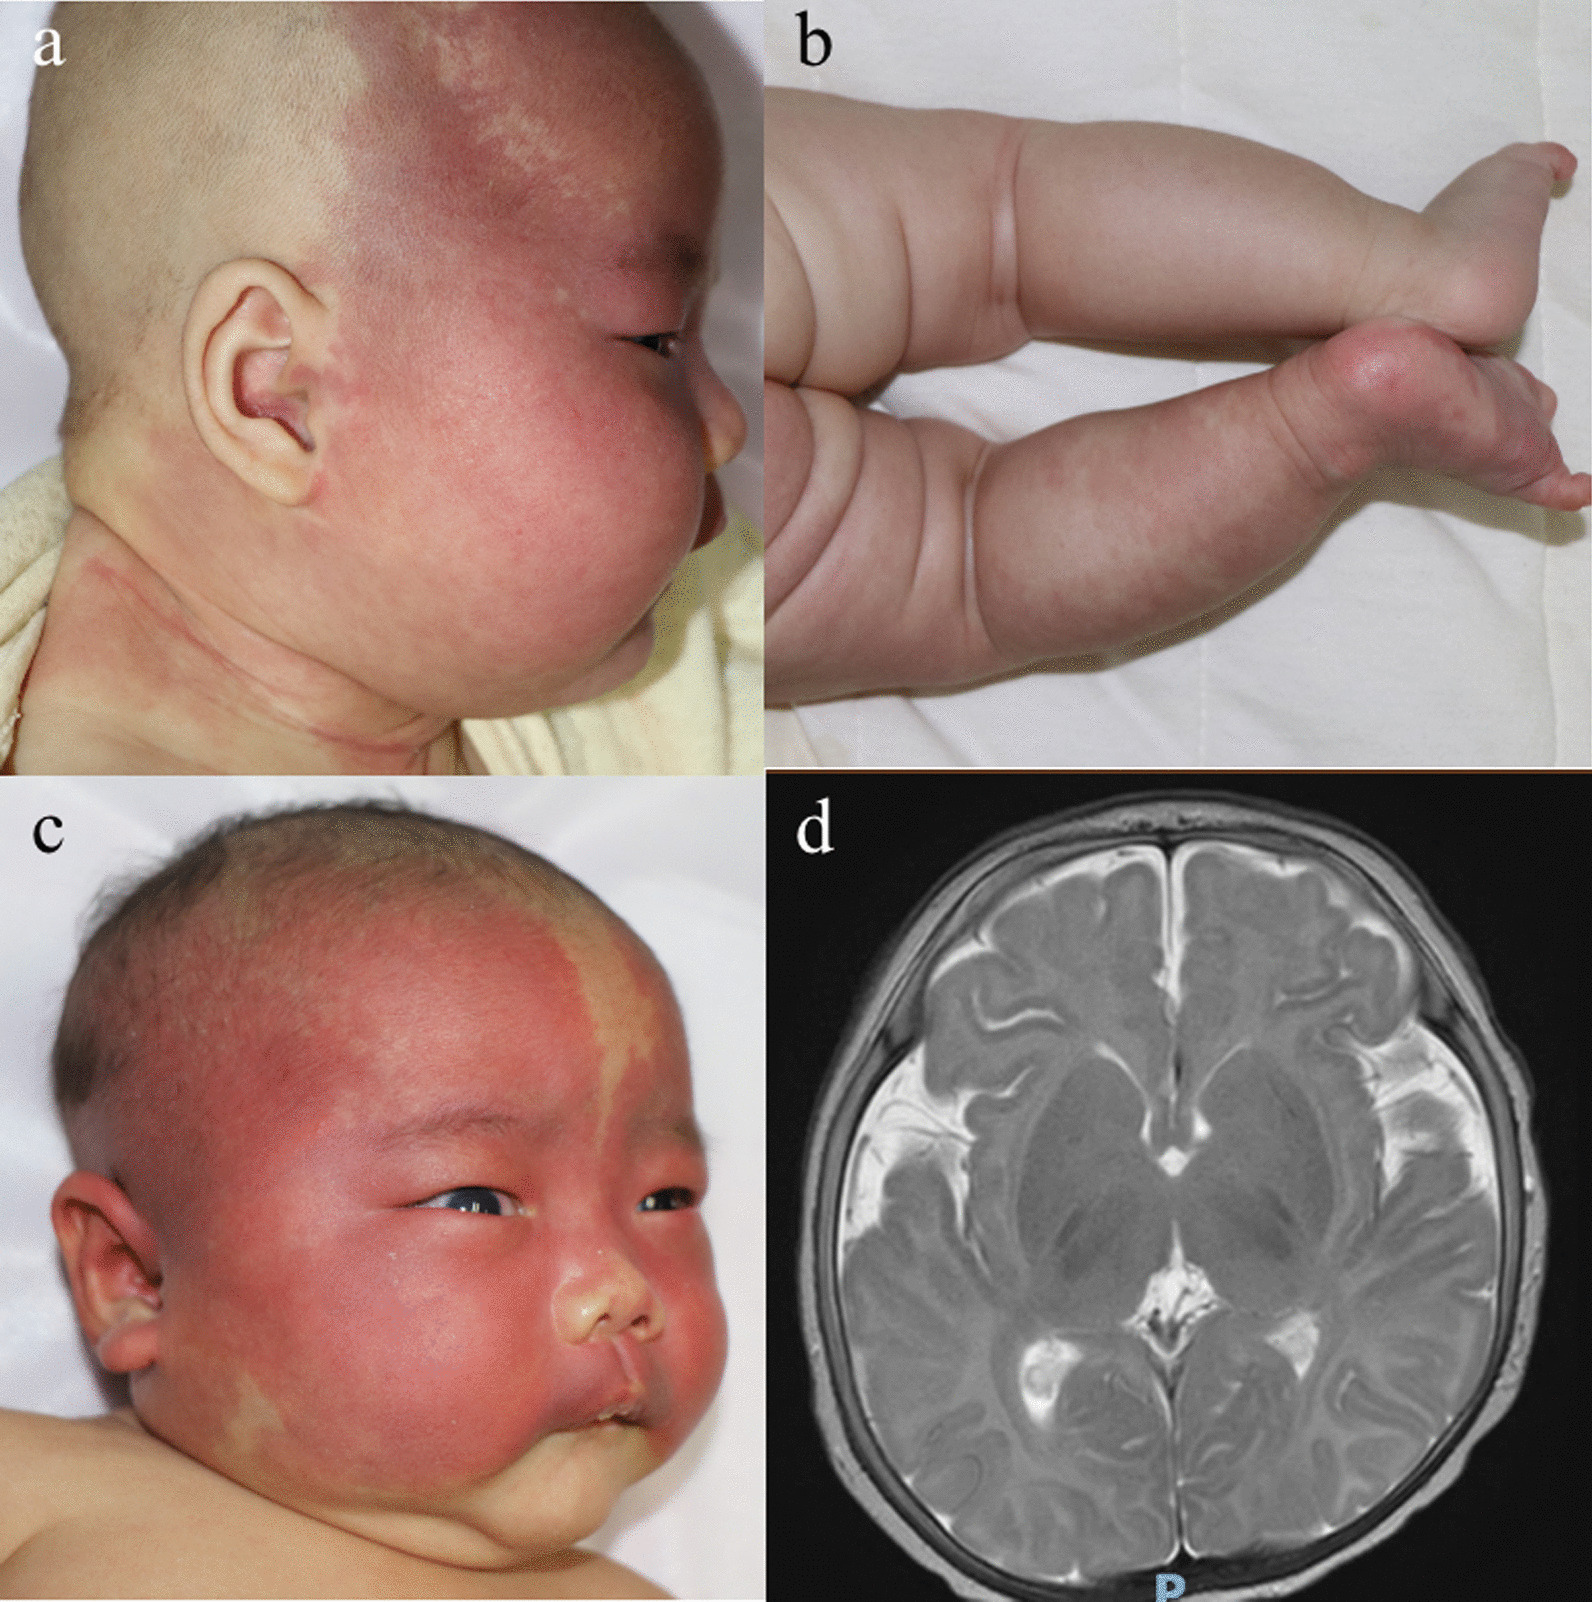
Clinical features of patients with PWS and SWS. a Patient P44, a 3-month-old girl with PWS and GNAQ c.548G > A;p.R183Q mutation. She had capillary malformations on the righ face and neck. She had normal intraocular pressure and no abnormalities in cerebral MRI. b Patient P41, a 3-month-old girl with PWS and GNAQ c.548G > A;p.R183Q mutation. She showed capillary malformation on the left lower limb and had symmetric low limbs. c Patient P36, a 2-month-old boy with SWS and GNAQ c.548G > A;p.R183Q. He had capillary malformation on bilateral lateral parts, right auricle and scalp with clear border, as well as pressure of fading and right eyeball protrusion. d MRI showed that outside the brain interval of bilateral temporal lobe were widened significantly with increased vascular shadows, and that some of them were circuity and dilated
